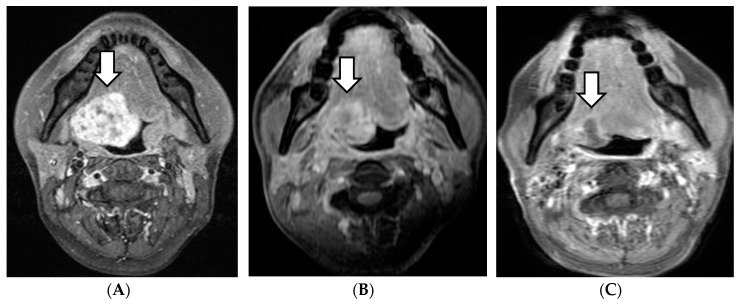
MRI of a patient, cT3N1MO case. The initial treatment effect was PR; thereafter, the tumor continued to shrink. (A) Before treatment. (B) After 3 months. (C) After 48 months. White arrow: initial or remaining tumor.
